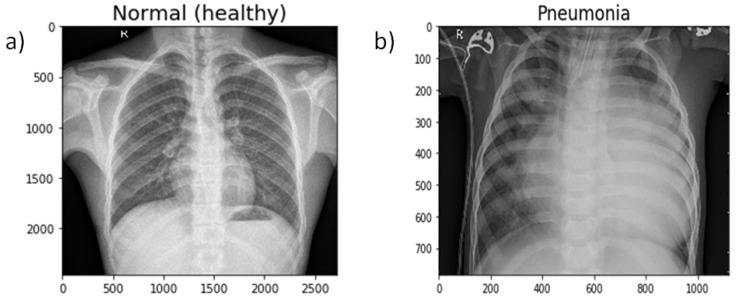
Chest Xray of (a) a healthy person and (b) a person suffering from pneumonia.
